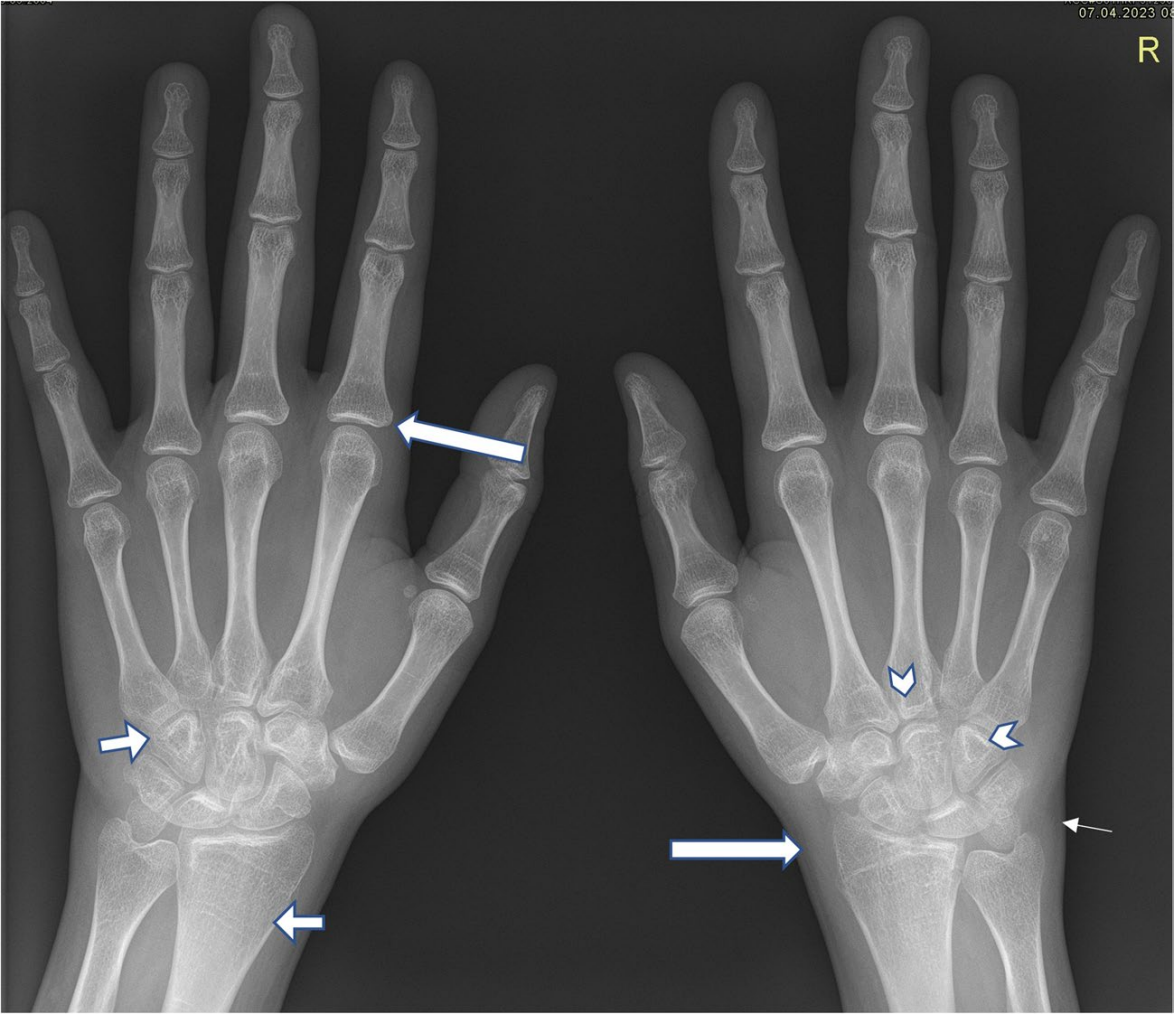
Anteroposterior radiographs of both hands in a 19-year-old young woman with a long history of aggressive seronegative polyarticular juvenile idiopathic arthritis, onset at the age of 7 years and with poor adherence to treatment. Images show mild bilateral periarticular osteoporosis (*long arrows*), growth arrest lines in the radial metaphysis and bone-in-bone appearance of carpal bones (*short arrows*), joint space narrowing of carpo-metacarpal joints and intercarpal bones, more pronounced on the right (*arrowheads*), and mild soft tissue edema around the right ulna (*thin arrow*)
